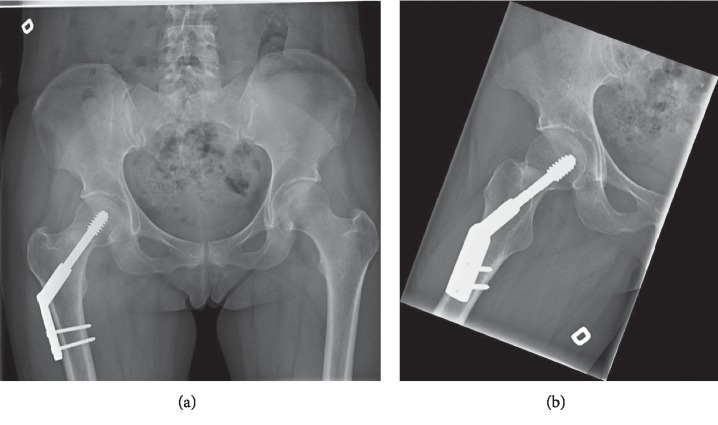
(a) AP pelvic X-ray and (b) lateral view of the right hip after dynamic hip screw (DHS), 6 weeks after surgery. The implant is in good position.
